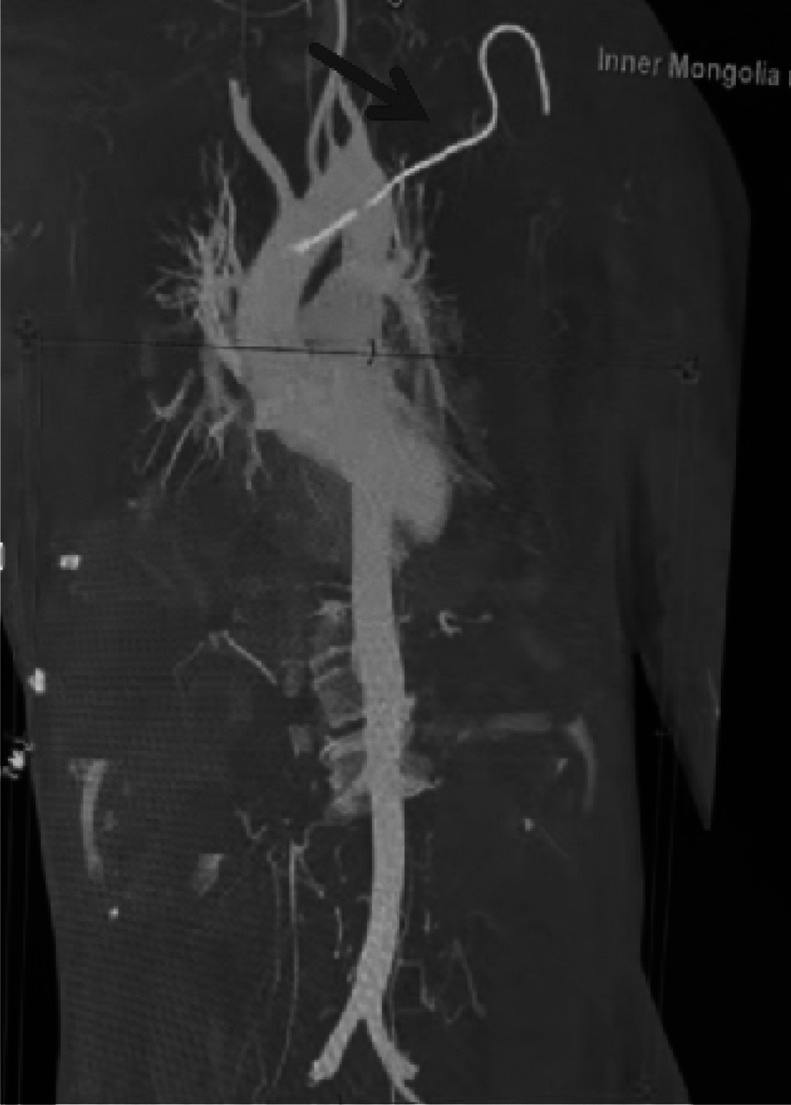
CT angiography of the entire aorta; the arrow indicates the aortic catheter placement.
